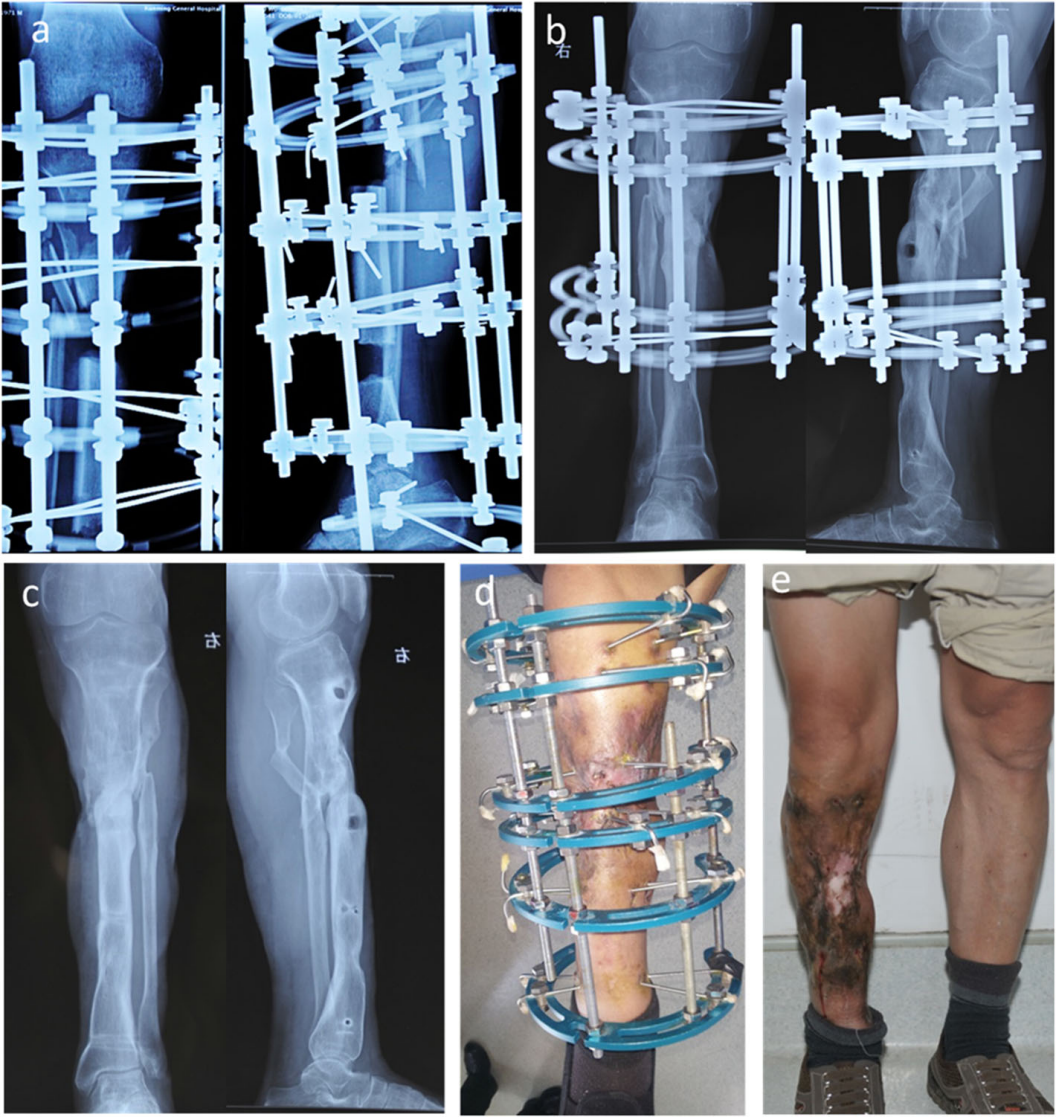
a Radiographs of distal and proximal osteotomy after meticulous debridement. The converged bone transport was performed on first day postoperative, and the proximal bone segment was transported at the rate of 1 mm/d, while the distal segment was transported at the speed of 0.6 mm/d, which was manipulated once in the morning and once in the evening. Two weeks later, the transport speed slowed down to 0.5 mm/d to 0.6 mm/d. b The docking site union and consolidation of regenerates were achieved 32 months after the operation. c Frame was removed with good bone result on 33 months after osteotomy. d-e Photographs of standing position with excellent functional result
